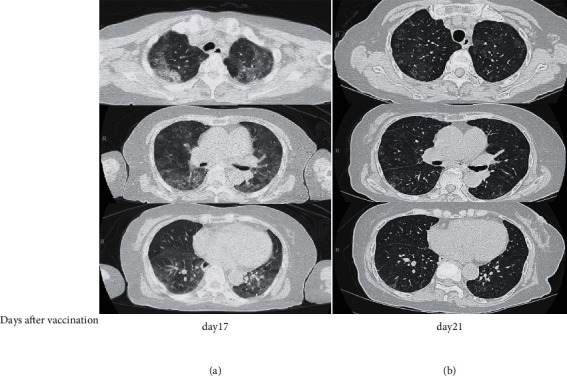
Chest computed tomography scans showing changes in the lung lesions. (a) CT scan performed on Day 17. (b) CT scan performed on Day 21. The second CT scan shows a clear improvement compared to the CT scan performed 4 days earlier. CT: computed tomography.
